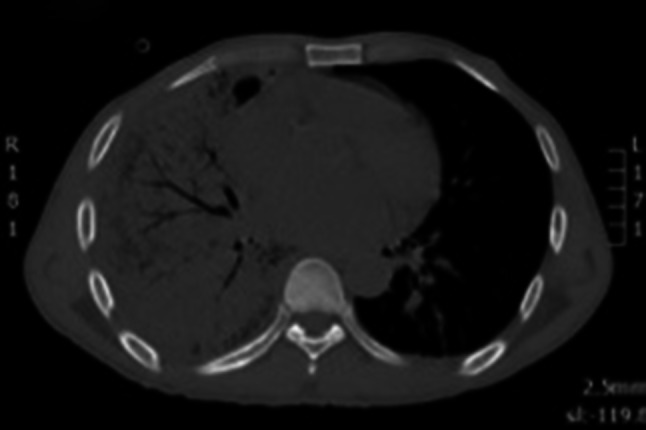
Lung CT scan showing right basal consolidation and air bronchograms
